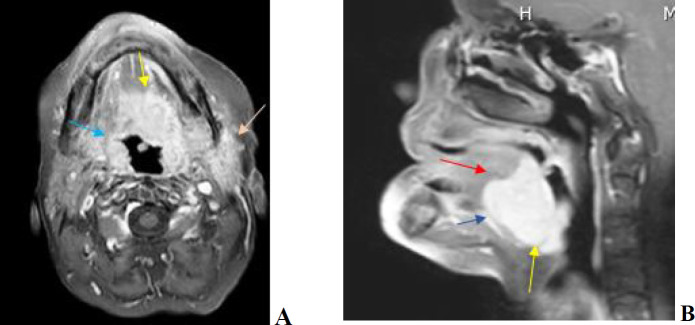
(a) MRI of the head and neck shows one large infiltrative enhancing mass arising from the left posterior aspect of the tongue (b), which crosses the midline and extends to the oropharyncous, left parapharyngeal space, left masticator, and parotid space
